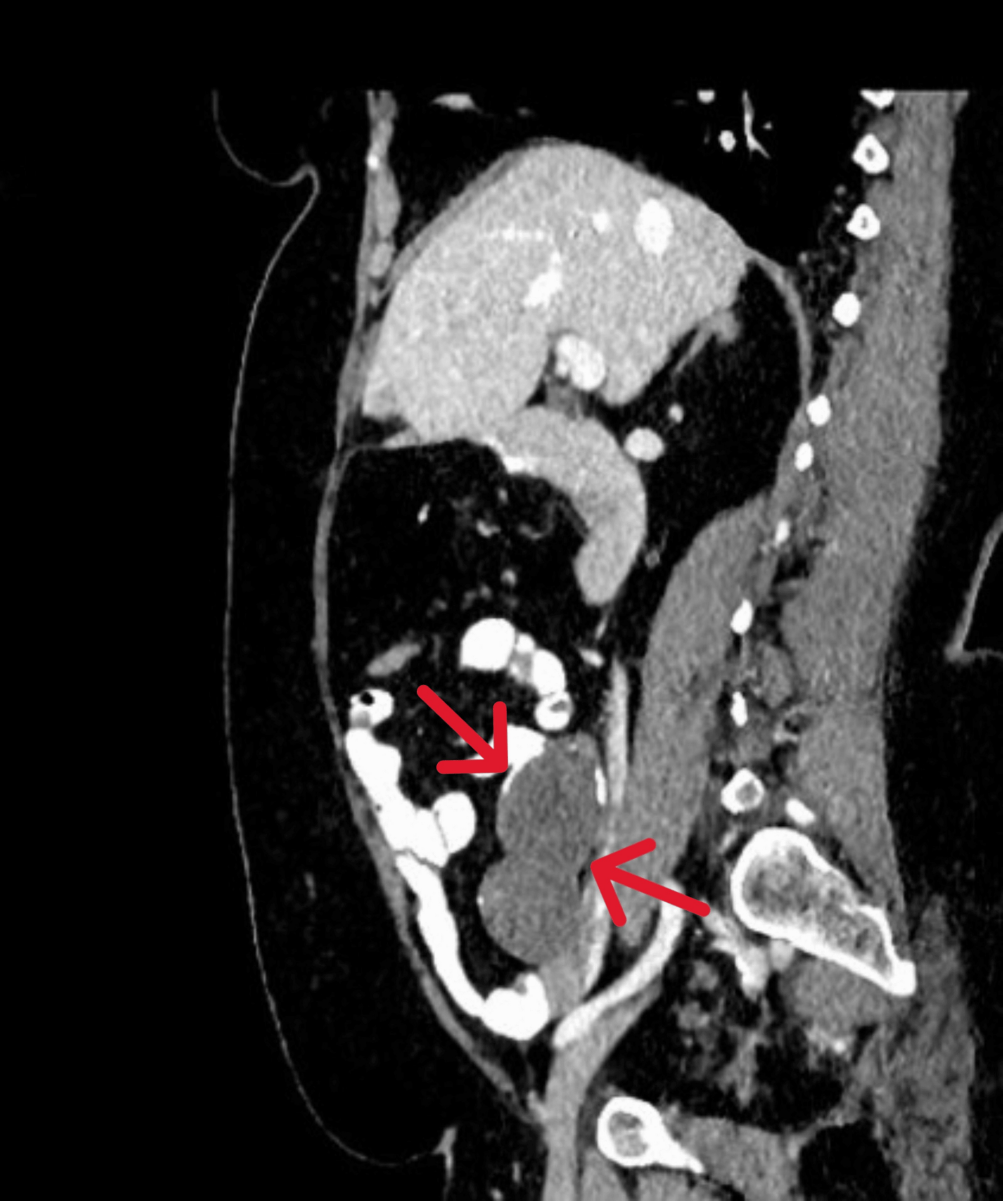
CT of the abdomen with contrast demonstrating hypodense appendiceal mucocele in sagittal view.
